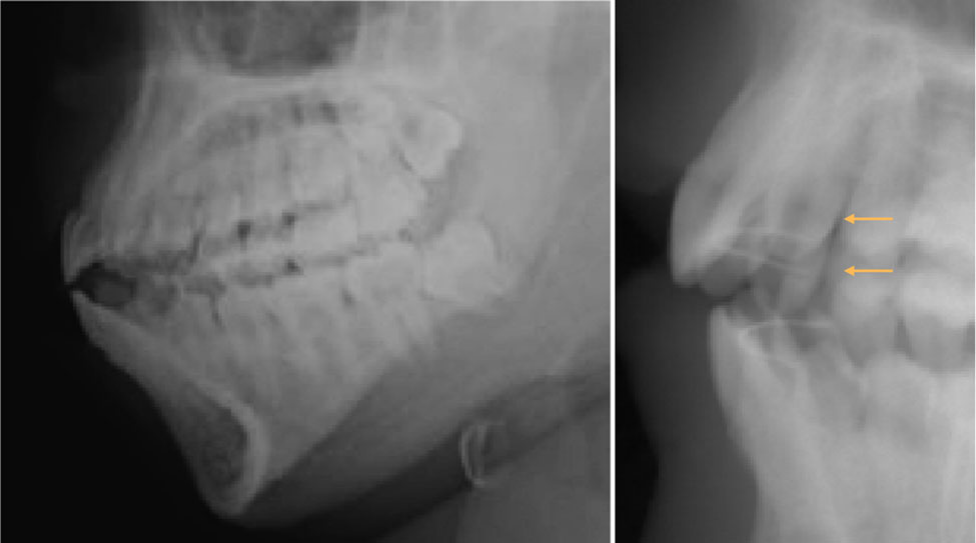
First-line postmortem 2 D radiographs, no dental care, complete arches few (mostly morphometric) data. On the right, there are only two orthodontic retainers (arrows).
